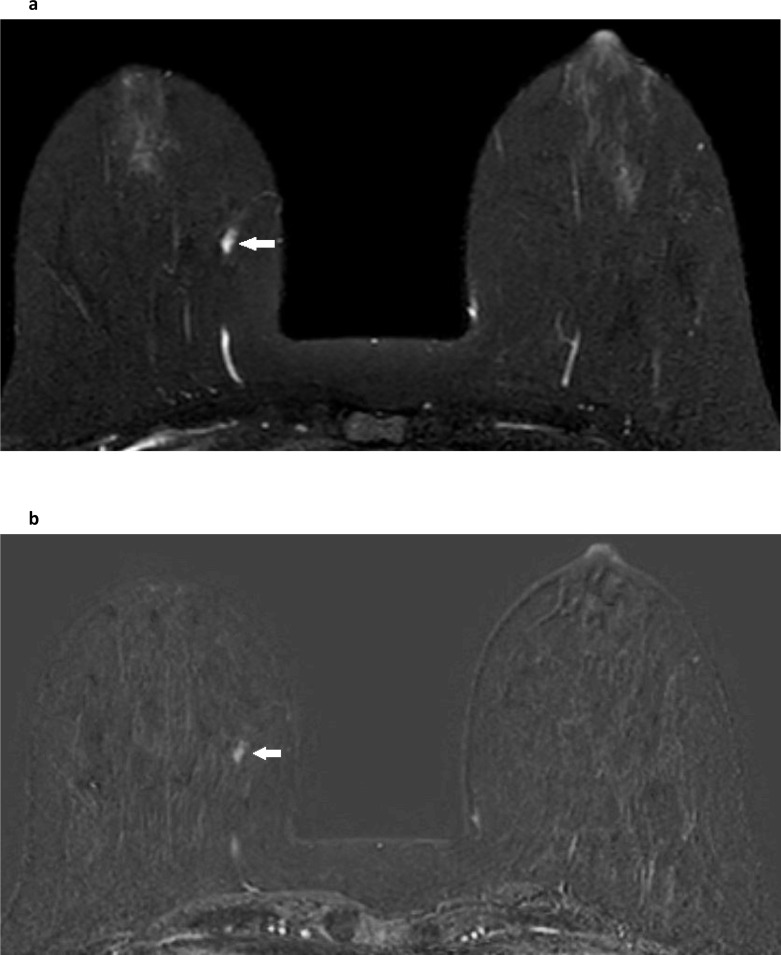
Fibromatosis. This breast MRI was performed for problem-solving following a symptomatic presentation of a suspicious-looking visible dent in the lower inner quadrant of the right breast. There was no palpable mass or other abnormality on examination and both mammography and ultrasound were normal. Fat-suppressed T2W imaging (a) showed a small hyperintense region (white arrow) in the medial right breast, and subtracted images from the dynamic series (b) showed a small focus of non-mass enhancement (white arrow). This was shown to represent fibromatosis at MRI-guided biopsy and at subsequent surgical excision.
